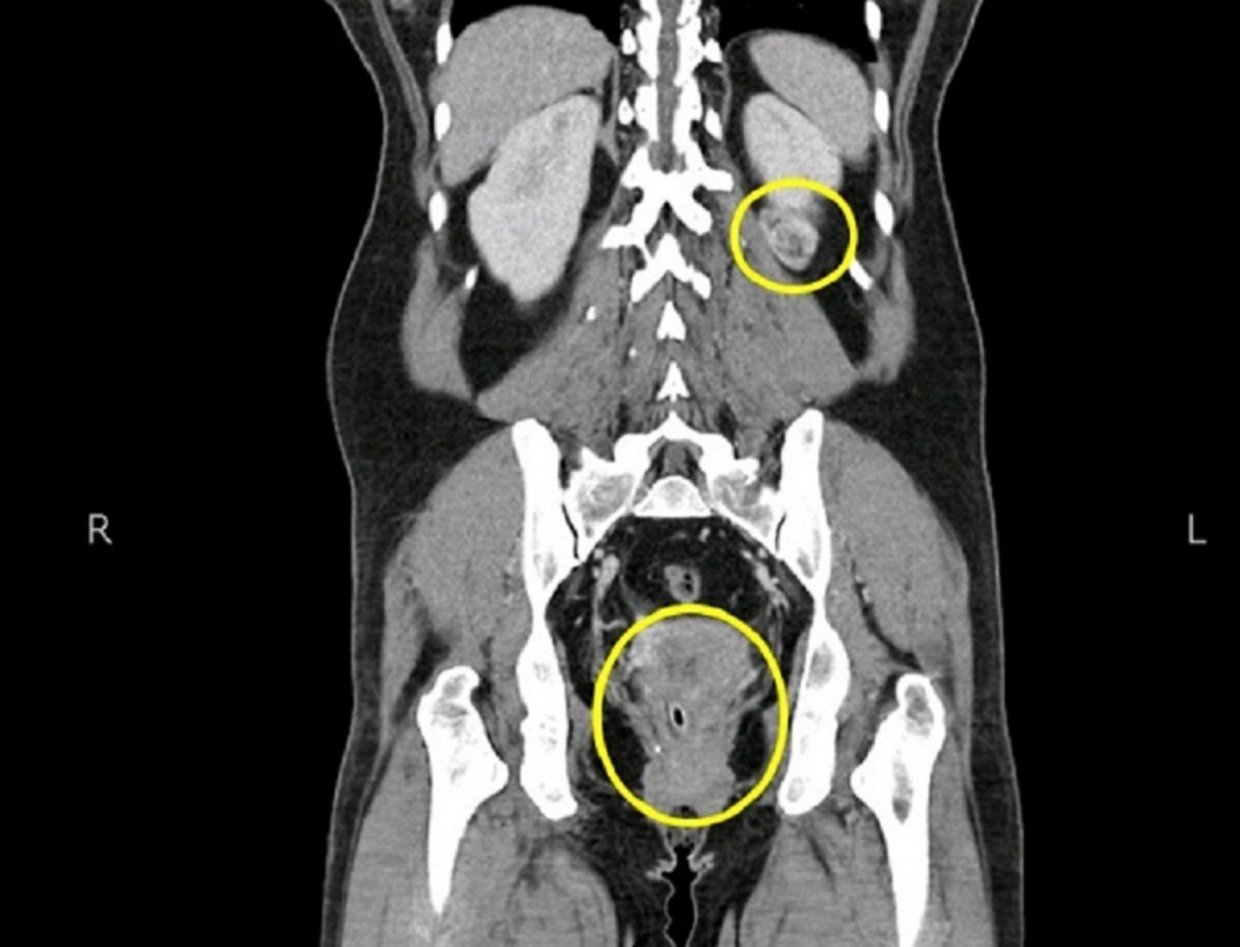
Computed tomography (CT) scan of the abdomen and pelvis showing a heterogeneous lesion in the cervix and the lower pole of the left kidney.
